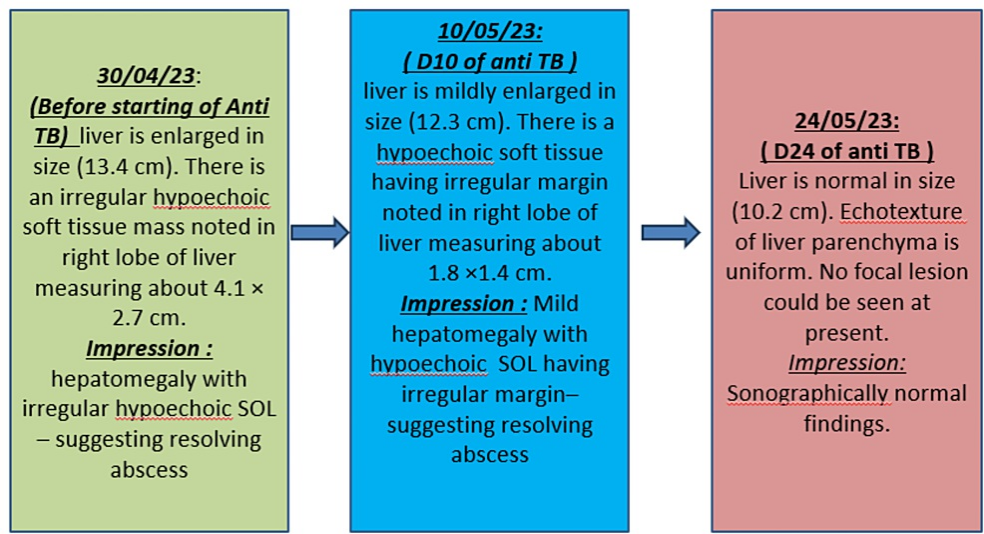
Serial ultrasonogram report summary with adequate treatment of tubercular abscess of the liver D10: Day 10; cm: Centimeter, D24: Day 24; SOL: Space occupying lesion
